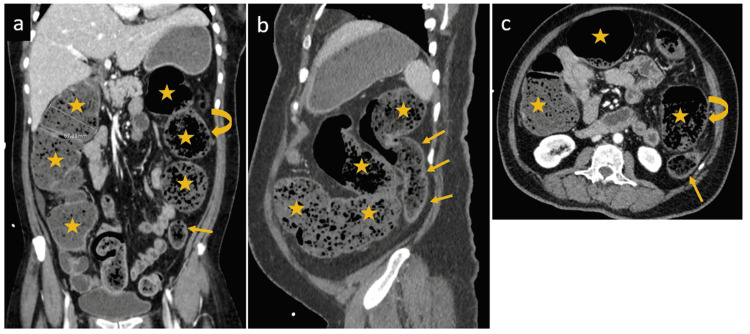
Initial contrast-enhanced abdominal CT scan. Coronal (A & B) and axial (C) images show diffuse dilation of the cecum, ascending and transverse colon (stars) up to the splenic flexure, followed by smooth tapering into the normal caliber descending and sigmoid colon (arrows) with the whole colon appearing impacted with feces. There is a short segment at the splenic flexure showing relative diffuse mild wall thickening with surrounding fat stranding (curved arrow)
